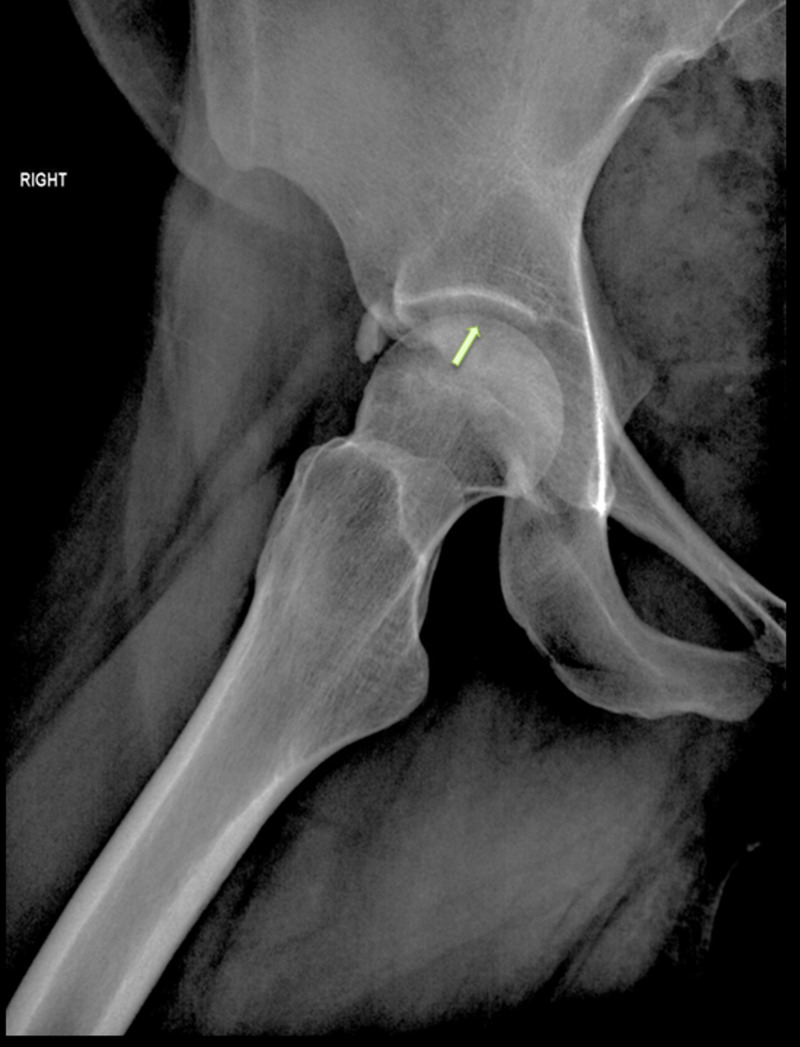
Lateral radiograph of the right hip showing a crescent sign
